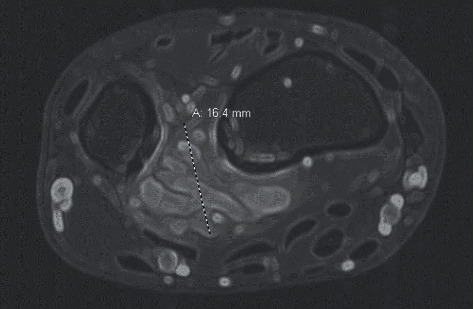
CT scan shows a lobulated 1.6 cm vascular mass in the pronator quadratus muscles along the interosseous membrane near the distal radioulnar joint.
